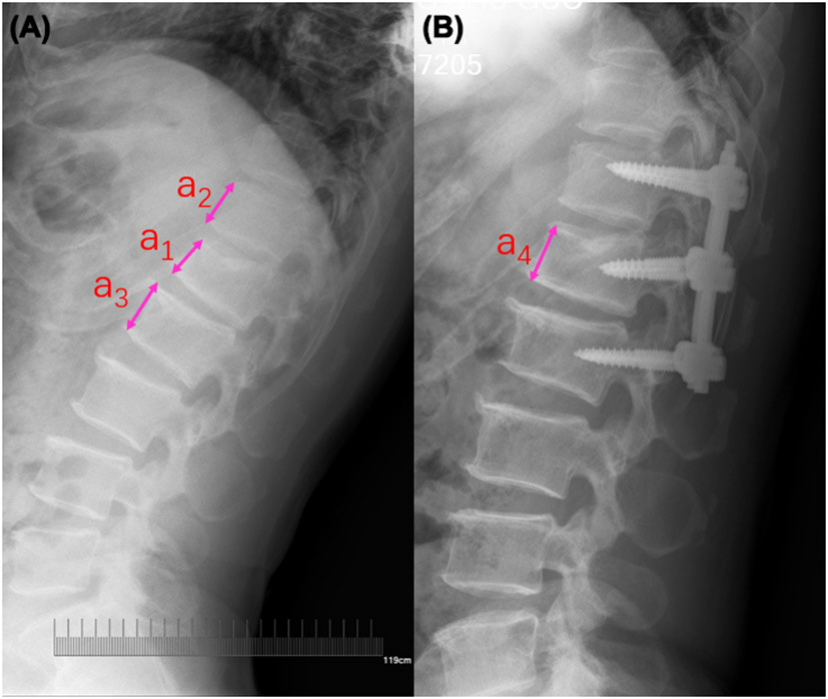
Radiographic measurement of the fractured vertebral body. (A) Pre-operative lateral radiograph; a_1_ is defined as the height of the fractured vertebra; the heights of the upper and lower anterior vertebrae that were adjacent to the fractured vertebra were presented as a_2_ and a_3_, respectively. (B) Post-operative lateral radiograph; a_4_ is defined as the height of the post-operative vertebra; the average height of a_2_ and a_3_ is calculated and defined as the predicted height of fractured vertebra (a_5_).
